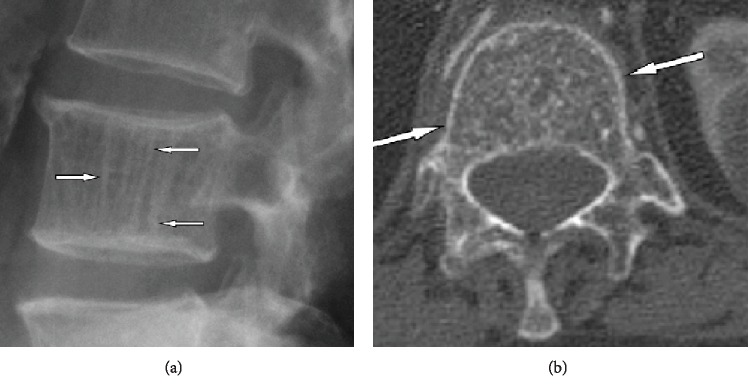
Lateral radiograph (a) exemplifying typical vertical striations due to thickened trabeculae. Axial CT scan (b) shows distinguishing polka-dot sign. Reprinted from [16].
